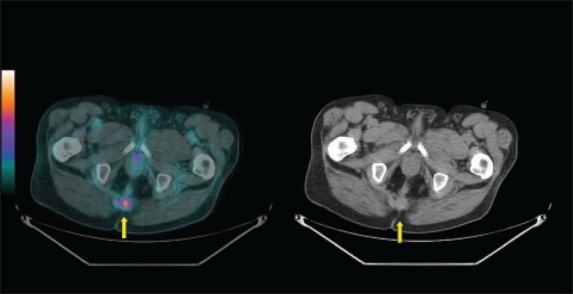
A 72-year-old man who had unexplained elevation of CEA during his follow-up after treatment of anorectal cancer. Fused (left panel) and CT (right panel) images of 18F-FDG PET/CT scan showed recurrent avid disease at the residual surgical bed soft tissue density (yellow arrow).
